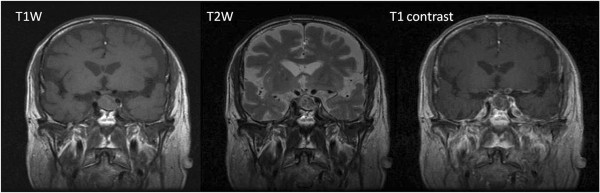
The enlarged pituitary gland with maximal size of 1.8 cm is noted in MRI examination. The signal characteristic of enlarged pituitary gland is non-specific, including hypointense signal on T2-weighted images and intermediate signal on T1-weighted images, with no water restriction on diffusion-weighted imaging. The most specific finding is non-enhancement of the pituitary gland, suggestive of infarction or so-called apoplexy.
